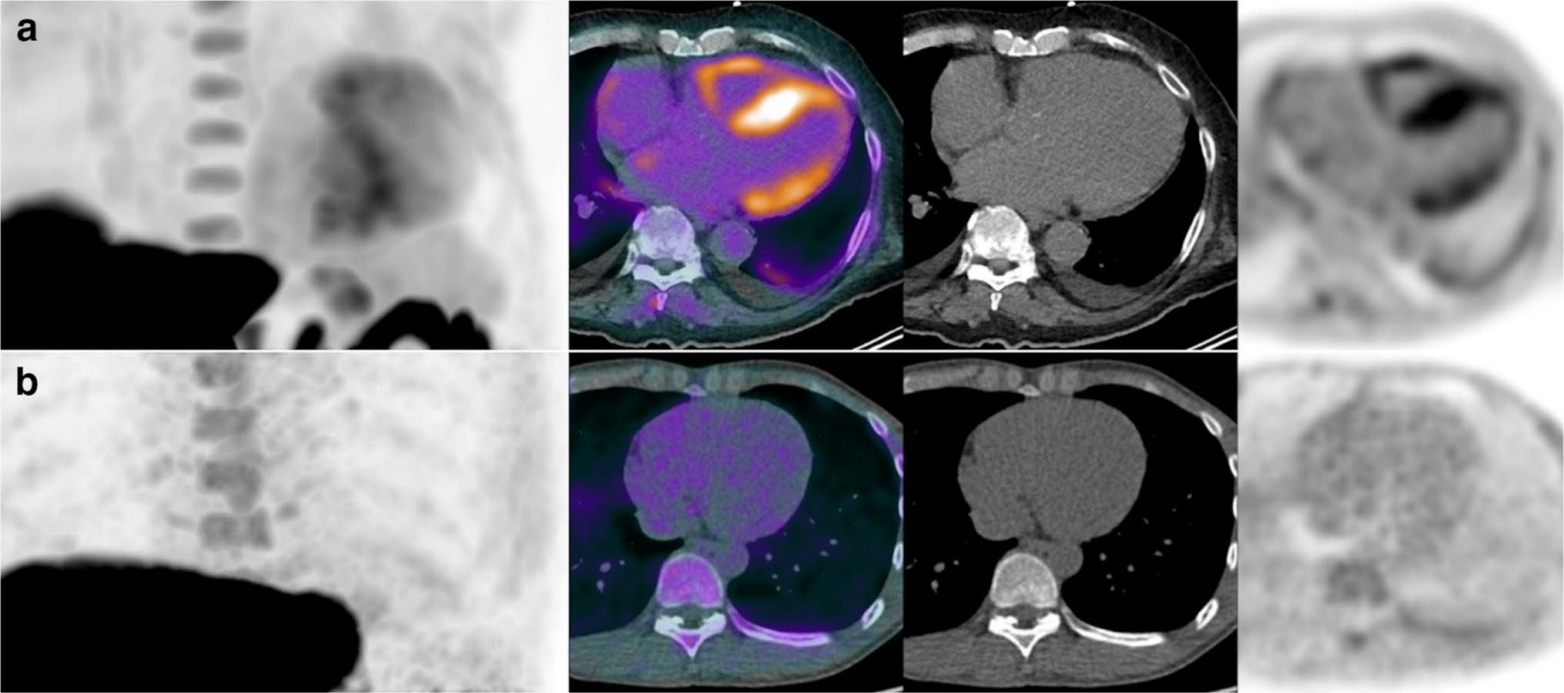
[^18^F]Flutemetamol imaging in subject with cardiac amyloidosis. a Showing high radiotracer uptake in the left and in the right myocardium, versus a representative control. b with no significant uptake in the myocardium, including in columns from the left to the right: maximum intensity projections (MIP), fused positron emission tomography (PET)/computed tomography (CT), CT, and PET. Reprinted with permission from [30]
